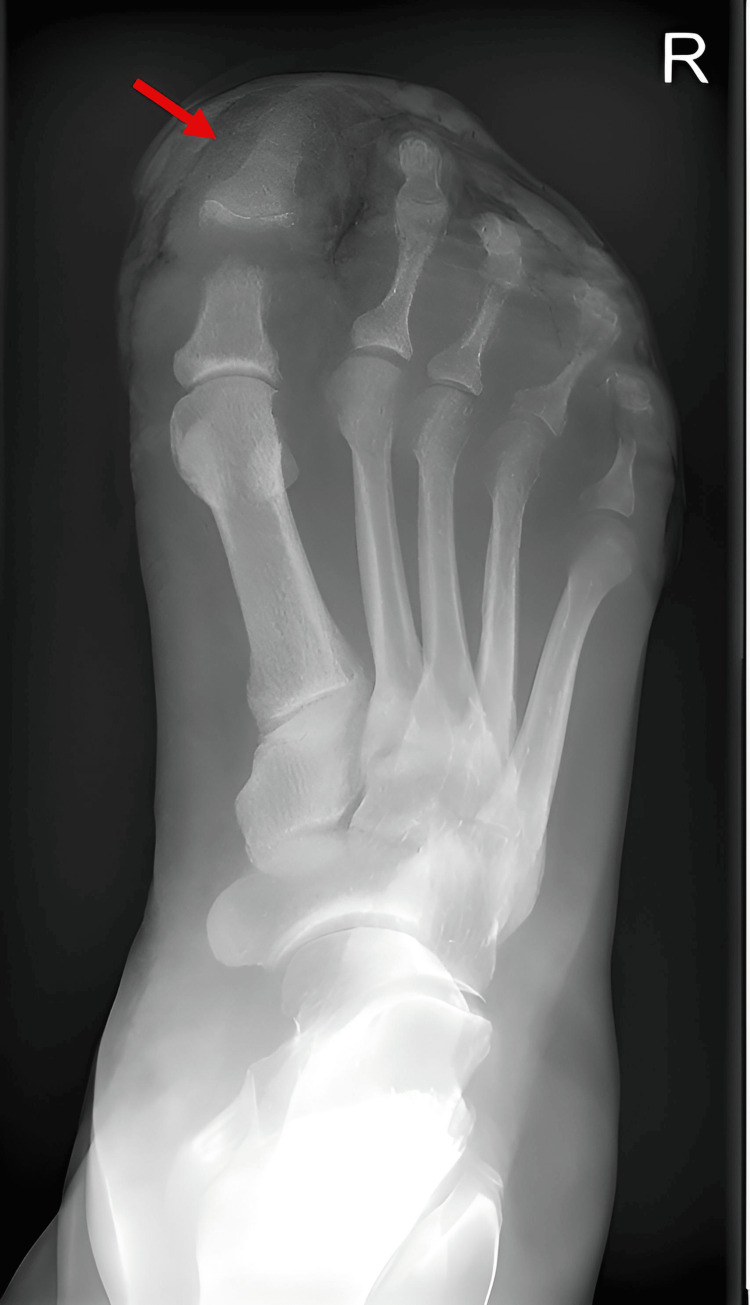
Follow-up radiograph two weeks later demonstrating progression of osteomyelitis in the great toe, with unchanged findings in the third and fifth toes.
